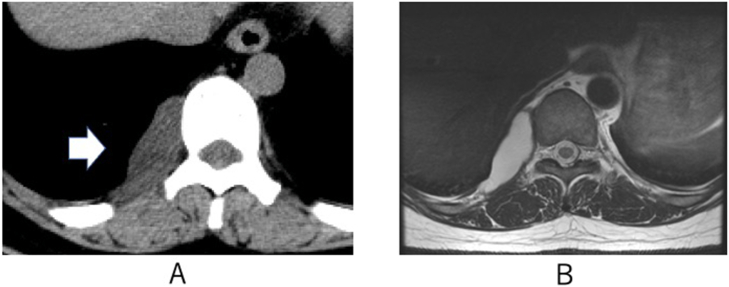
A: Chest computed tomography revealed a posterior mediastinal mass (arrow) measuring 4.2 × 1.8 × 3.2 cm close to the tenth thoracic vertebra. B: Chest magnetic resonance imaging revealed hypo-intensity on T1-weighted images and hyper-intensity on T2-weighted images.
